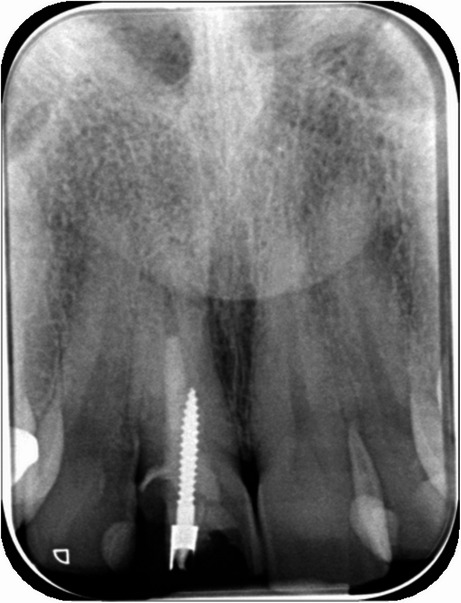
Pre-operative periapical X-ray
